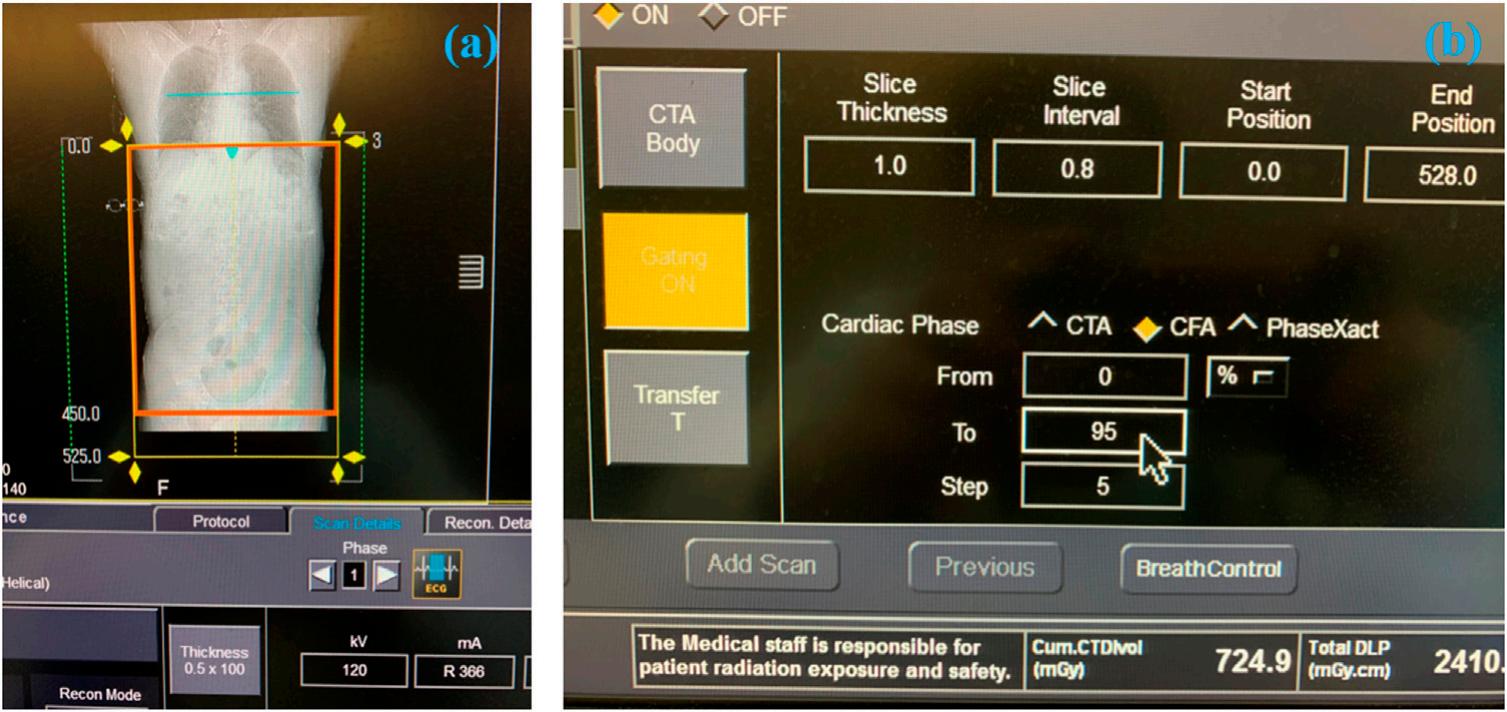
The process of obtaining the 4D CT image data. (A) The selected scanning range of AAA. (B) Parameters setting during scanning.
